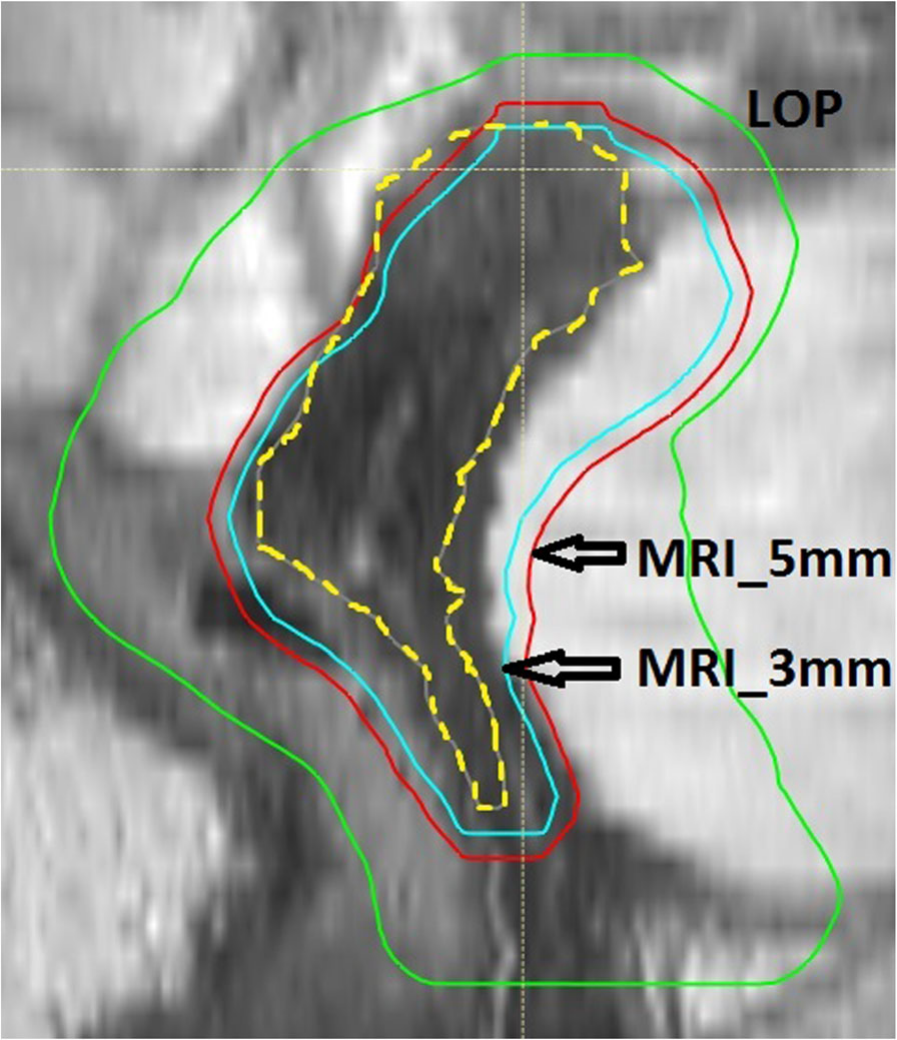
PTV of the selected plan in the LOP (green), and the PTVs of the MRI_5mm (red) and the MRI_3mm (blue) strategy overlaid on a transversal MRI. The low-risk CTV on the transversal MRI is indicated by the dashed yellow line. In this example, due to intrafraction bladder filling, the coverage of the low-risk CTV was insufficient for the MRI_3mm strategy (D_98%_ < 95%), while it was sufficient for the MRI_5mm and LOP strategy
